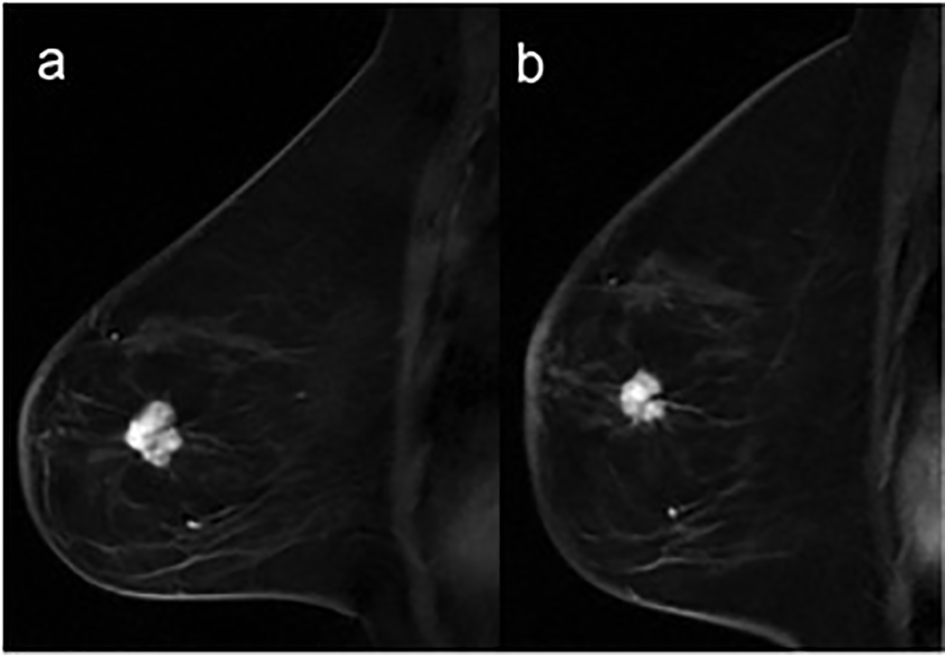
Breast MRI of a 57-year-old sarcopenic woman (SMI = 34.3 cm^2^/m^2^; SMI < 40 cm^2^/m^2^ = sarcopenia) with invasive ductal carcinoma of the left breast. Sagittal post-contrast T1-weighted images, before (A) and after (B) 6 months of treatment shows a substantial stability in the size of the lesion located in the lower-outer quadrant of the left breast.
